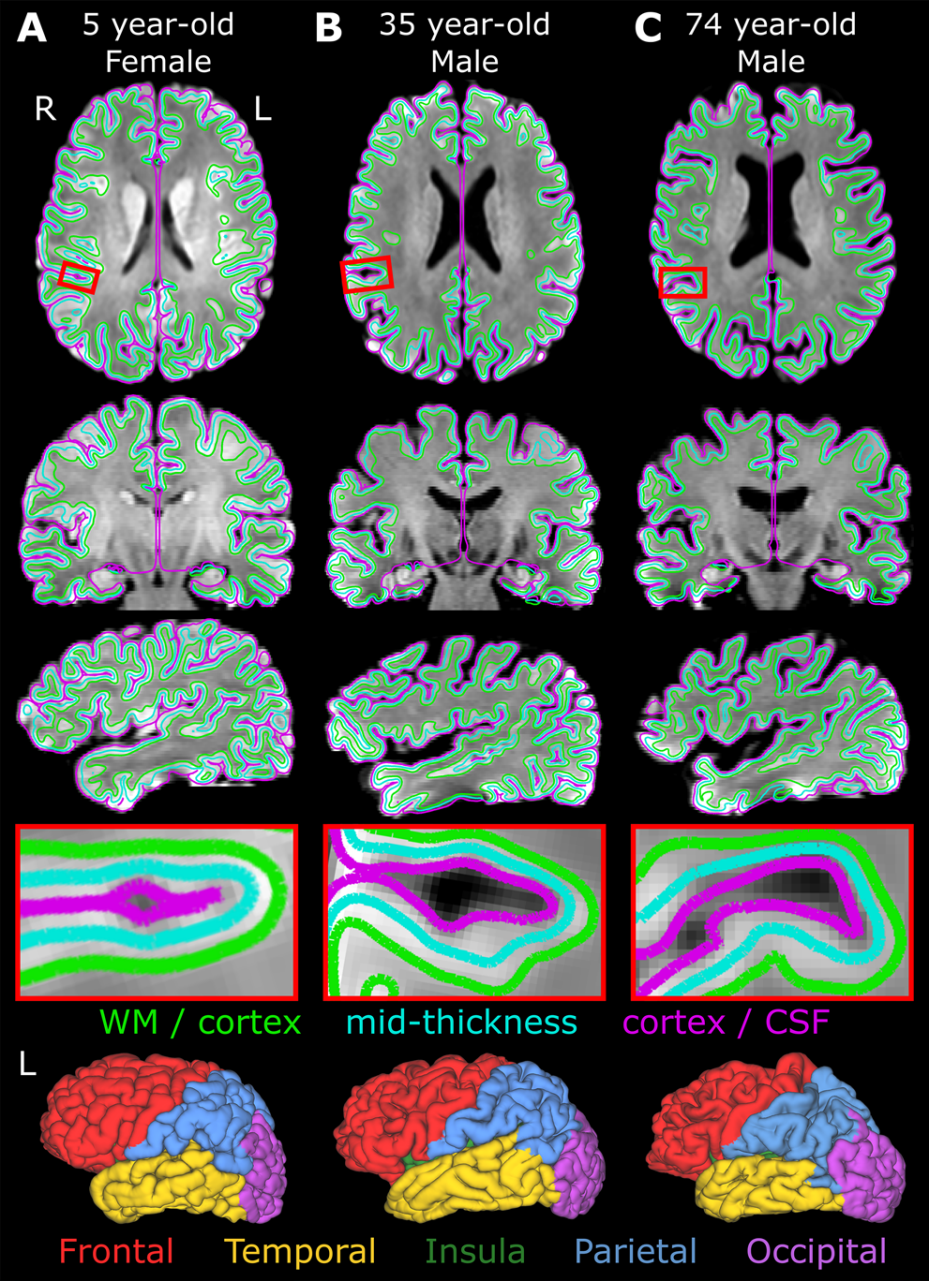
Examples of the diffusion image only automated segmentation to delineate the cortical surfaces (magenta - cortex/CSF, cyan - midthickness, and green - WM/cortex), as shown on the mean 1.5 mm isotropic DWI in three orthogonal planes for three participants (A–C) representing different ages across the lifespan. The red boxes show zoomed in regions. The bottom row shows their five lobe segmentations in the left hemisphere (cortex/CSF surface is shown).
